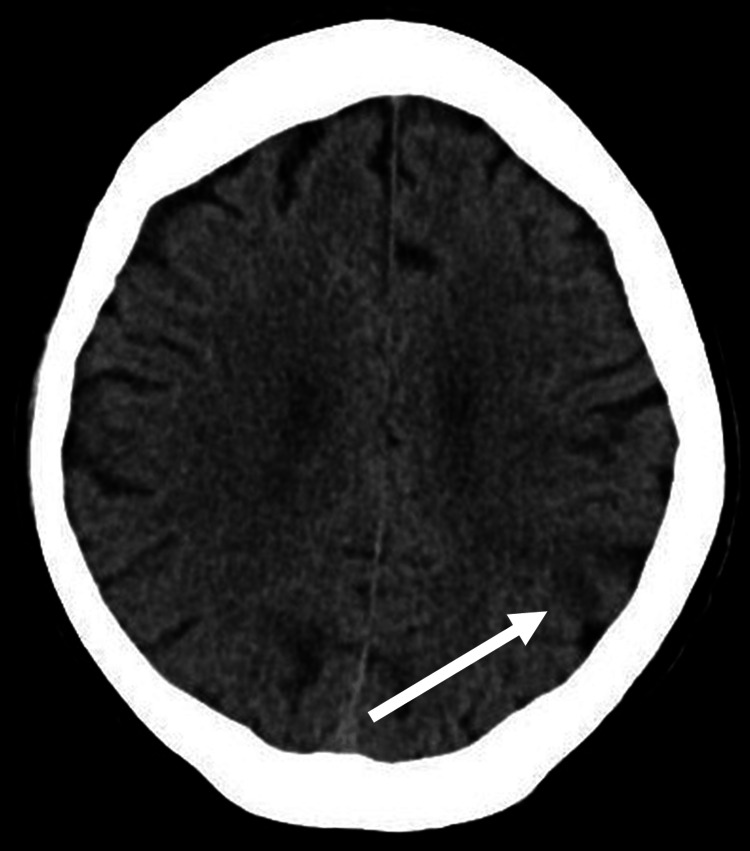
CT head showing acute infarct of the left parietal lobe. The arrow indicates the area of hypodensity indicative of an acute stroke. Axial non-contrast CT image showing an area of hypodensity in the left parietal lobe, consistent with an acute ischaemic infarct in the middle cerebral artery territory. (Image obtained from the hospital PACS system with patient consent.) The arrow indicates the area of hypodensity indicative of an acute stroke. PACS: Primary Agricultural Credit Societies
